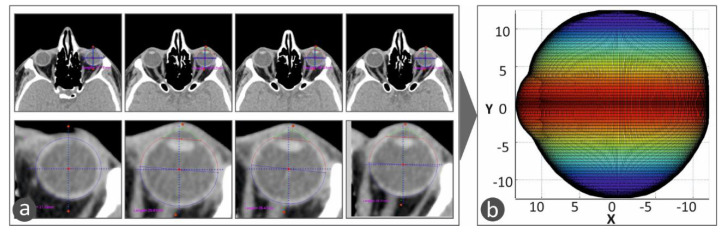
The 3D model of the human eye in the developed software. A color scale was used in the vertical direction to show the human eye height vs. the change in the human eye section size in the horizontal direction: (a) CT scan images in which the perimeter of the eye is determined and acquired, (b) 3D geometric model of the eye determined from the CT scan images and represented with a color scale
